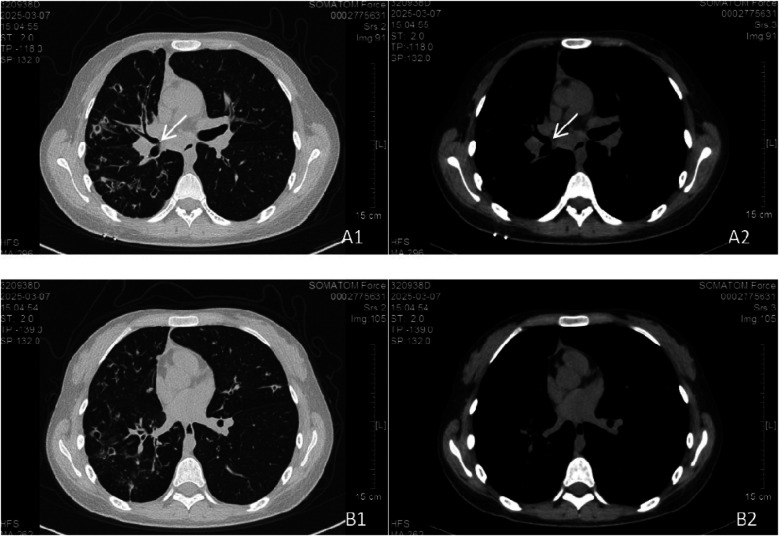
Chest CT scan at follow-up. The bilateral pulmonary lesions had progressed compared to Figure 4. Bronchiectasis with cavitation in the right lung increased in both number and size. Lesions in the left lung also increased. (A1, A2) Persistent parietal nodules adjacent to the right middle bronchus. (B1, B2) Parietal nodules in the basal segment of the left lower lobe were not visualized, possibly due to CT slice thickness or scan level.
